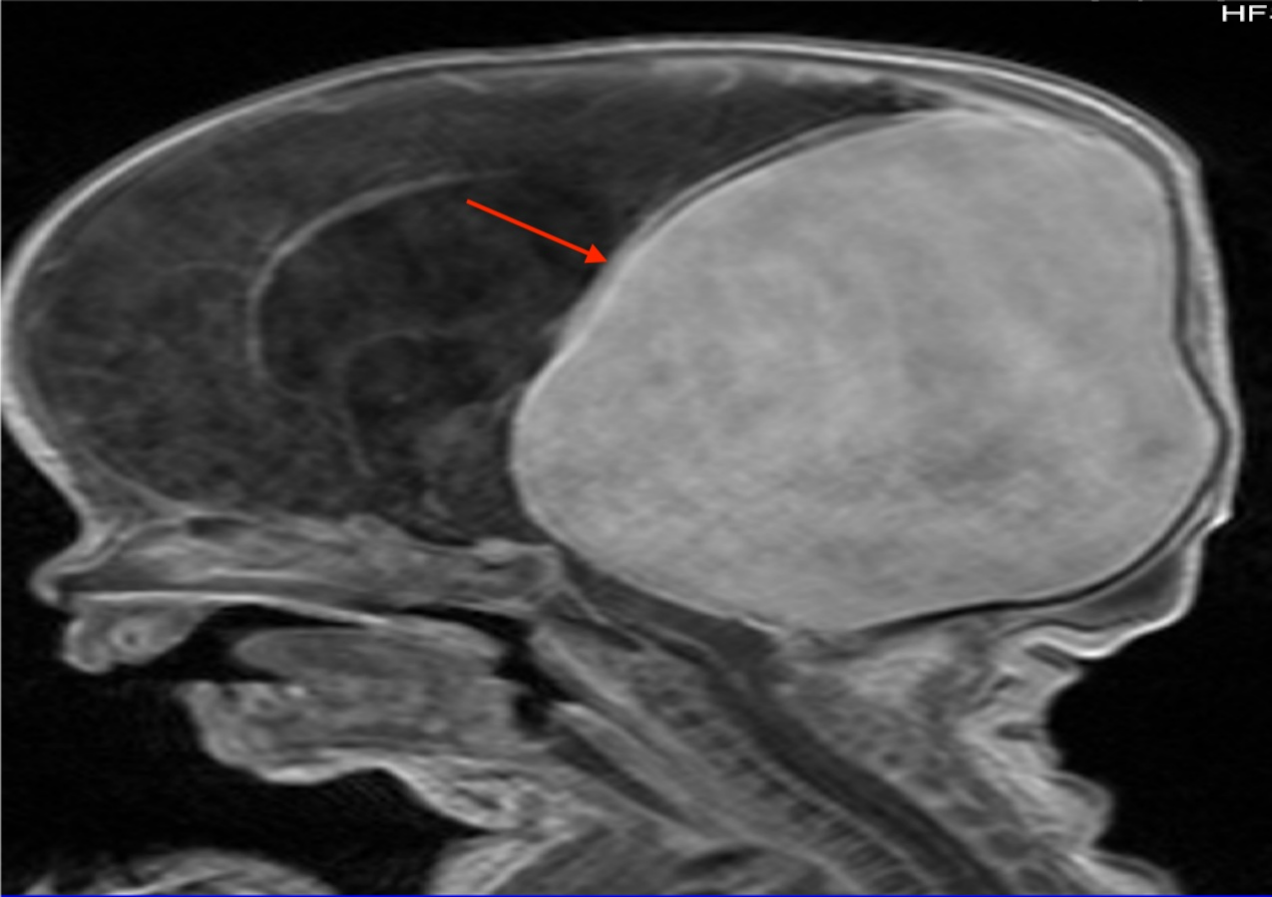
Post-contrasted MRI of a patient with a large VGAM (red arrow) seen in Figures 2 and 3 MRI: magnetic resonance imaging; VGAM: vein of Galen aneurysmal malformation.
